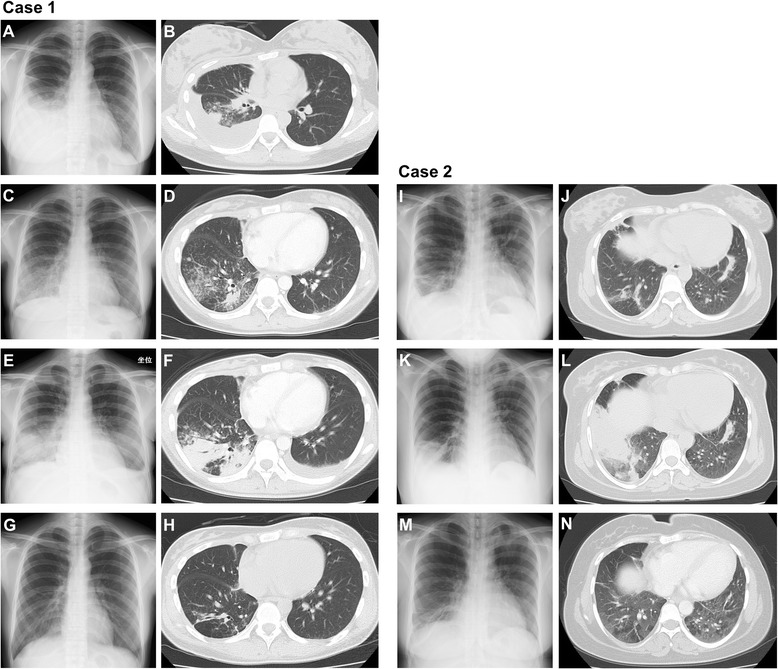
Time course of chest X-ray and CT findings of case 1 (a–h) and case 2 (i–n). a and b, Right pleural effusion and homonymous lung infiltration 1 month after delivery. c and d, Residual lung infiltration without effusion 4 months after delivery. e and f, Worsening of pre-existing lung infiltrations with new contralateral effusion 4 weeks after initiation of the antituberculosis treatment. g and h, Disappearance of effusion and improvement of lung infiltrations after a course of chemotherapy (2HREZ/7HR). i and j, Multiple infiltrative shadows in lungs 6 months after delivery. k and l, Worsening of infiltrations in the right lung 10 weeks after initiation of the antituberculosis treatment. m and n, Improvement in lung infiltrations after a course of chemotherapy (2HREZ/7HR)
